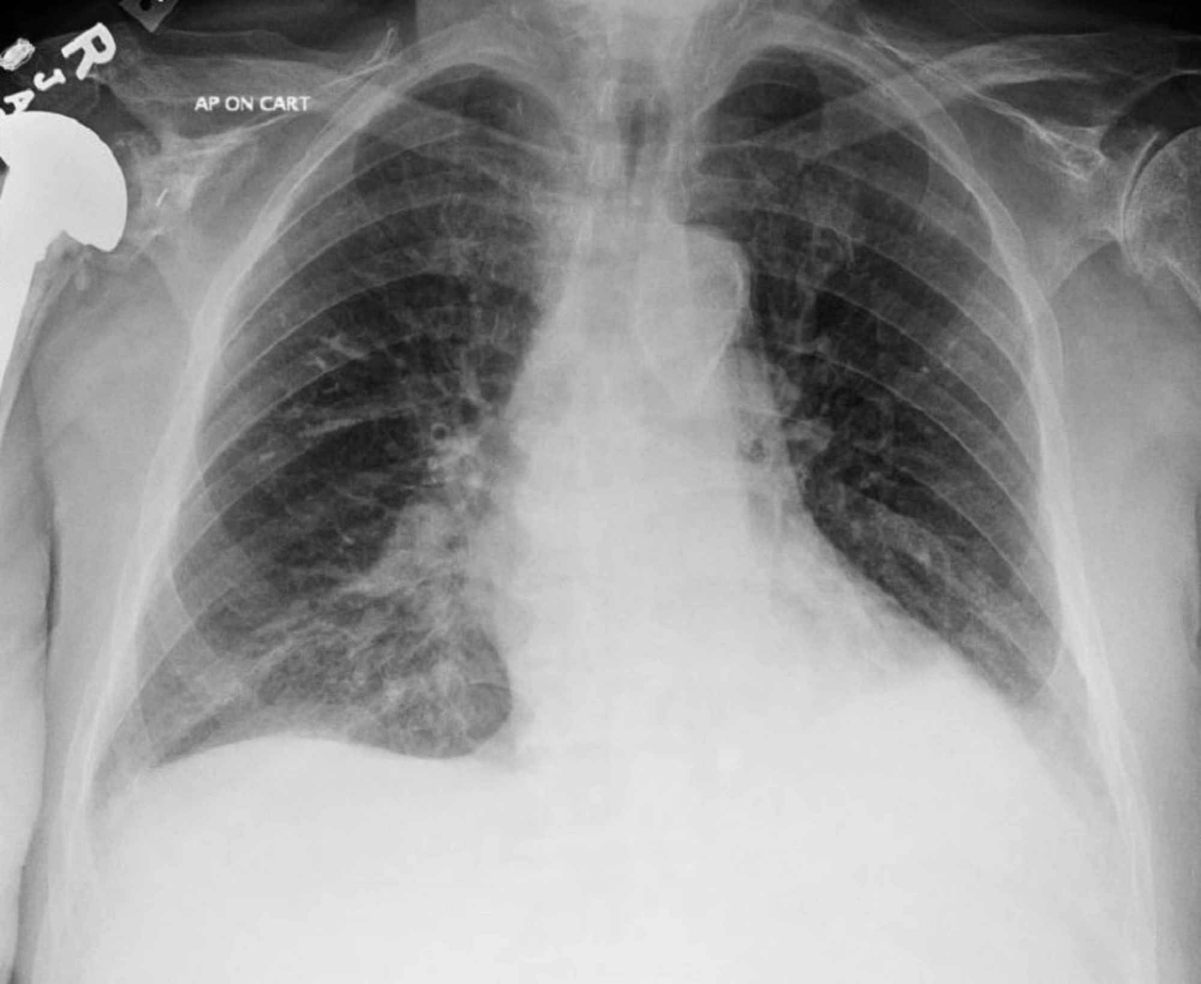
Chest X-ray was negative for any acute findings
